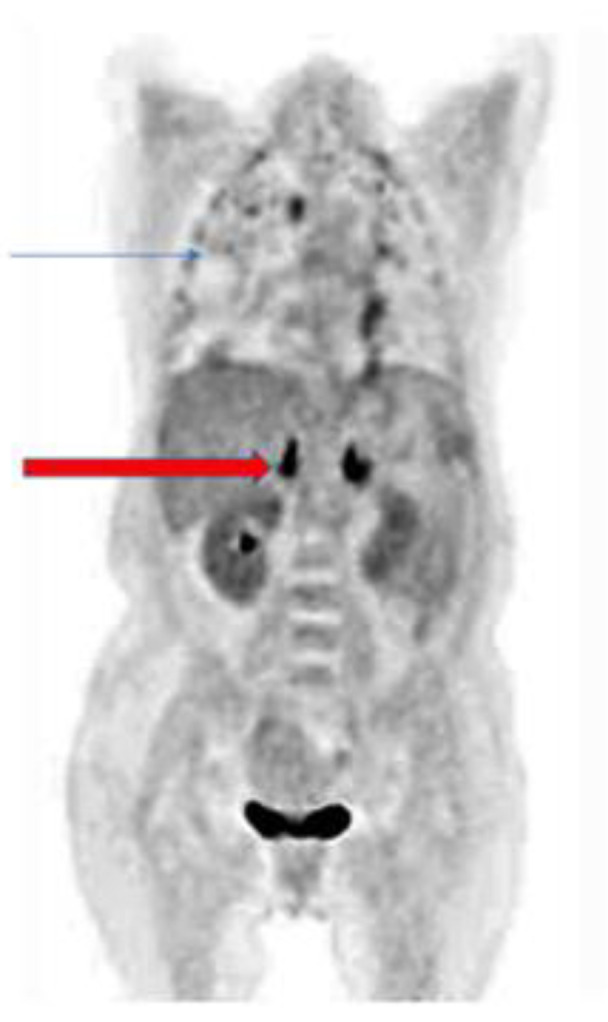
Patient with pulmonary cryptococcosis (blue arrow) with bilateral intense hypermetabolic uptake on coronal slice of ^18^F-FDG PET due to adrenal involvement (red arrow).
